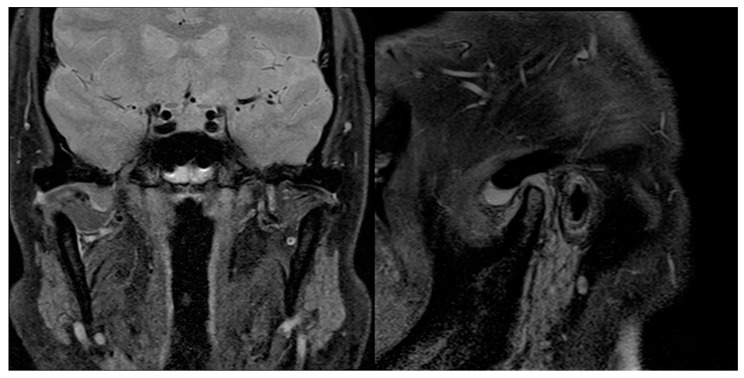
Preoperative sagittal and coronal MRI scans showing, on the right, TMJ joint effusion and LBs, but no bony involvement.
